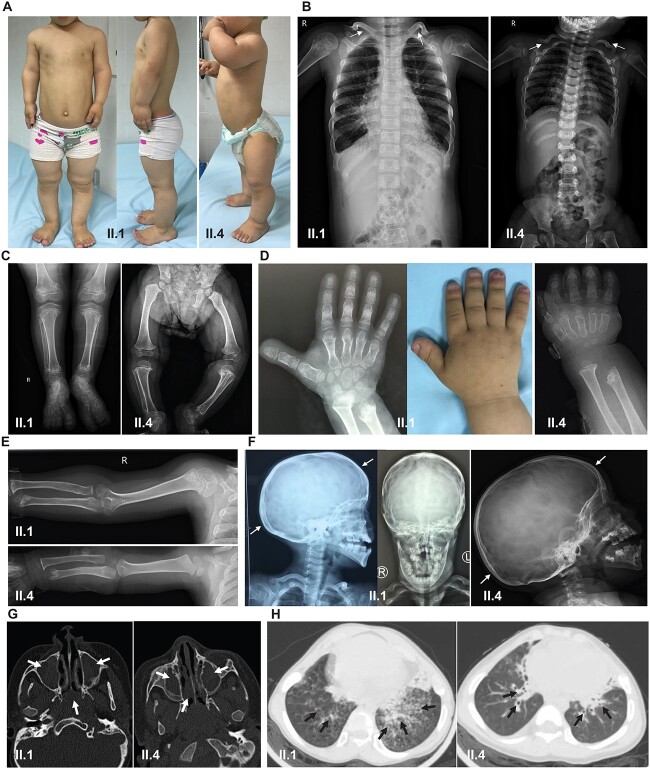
Clinical characteristics of individuals with biallelic *IFT74* exon 2 deletion mutations indicating both skeletal dysplasia and respiratory symptoms. (A) Both affected children II.1 (aged 8) and II.4 (aged 2) showed abnormal bone development with a short stature characterized by shortened arms and legs reminiscent of certain skeletal ciliopathies, bowing of the legs, which was more prominent in the older sibling II.1, and spine curvature (lordosis) more evident in II.4. Chest X-rays showed malformed clavicles (arrows) and narrowing of the ribs and thorax, the thorax in II.4 being slightly more narrowed, and bell shaped (B). X-rays show the shortened long bones with widened ends of the long bones (epiphyses) affecting both legs (C) and arms (E), and mildly shortened digits (brachydactyly) without polydactyly (extra digits) of the hands and feet (A, D) affecting both siblings. Cranial X-ray showed that dolichocephaly also affected the skull in both siblings, i.e. elongated skull diameter, front to back as indicated by arrows (F). (G) Both affected siblings II.1 and II.4 had symptoms of motile cilia disease shown in axial-view CT scans demonstrating swollen, inflamed sinuses and mucus blockage (arrows). (H) Chest X-ray of II.1 and II.4 shows features of bronchiectasis including enlarged airways and bronchi with mucous accumulations (arrows).
